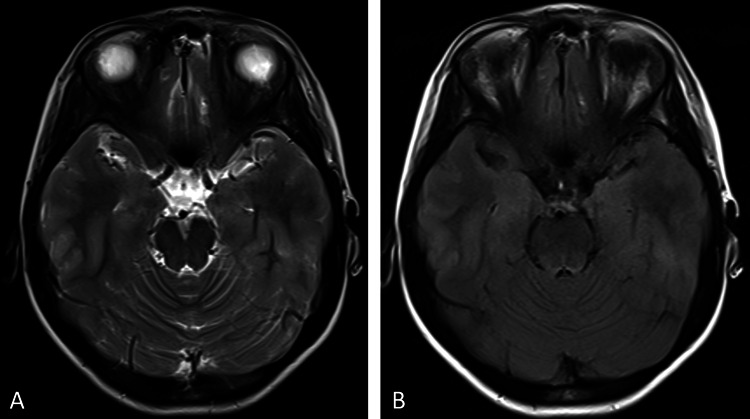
Re-scan images of Case 3 Artifact resolution in (A) T2 and (B) T2FLAIR sequences. T1 was not re-scanned. FLAIR: Fluid-attenuated inversion recovery.
